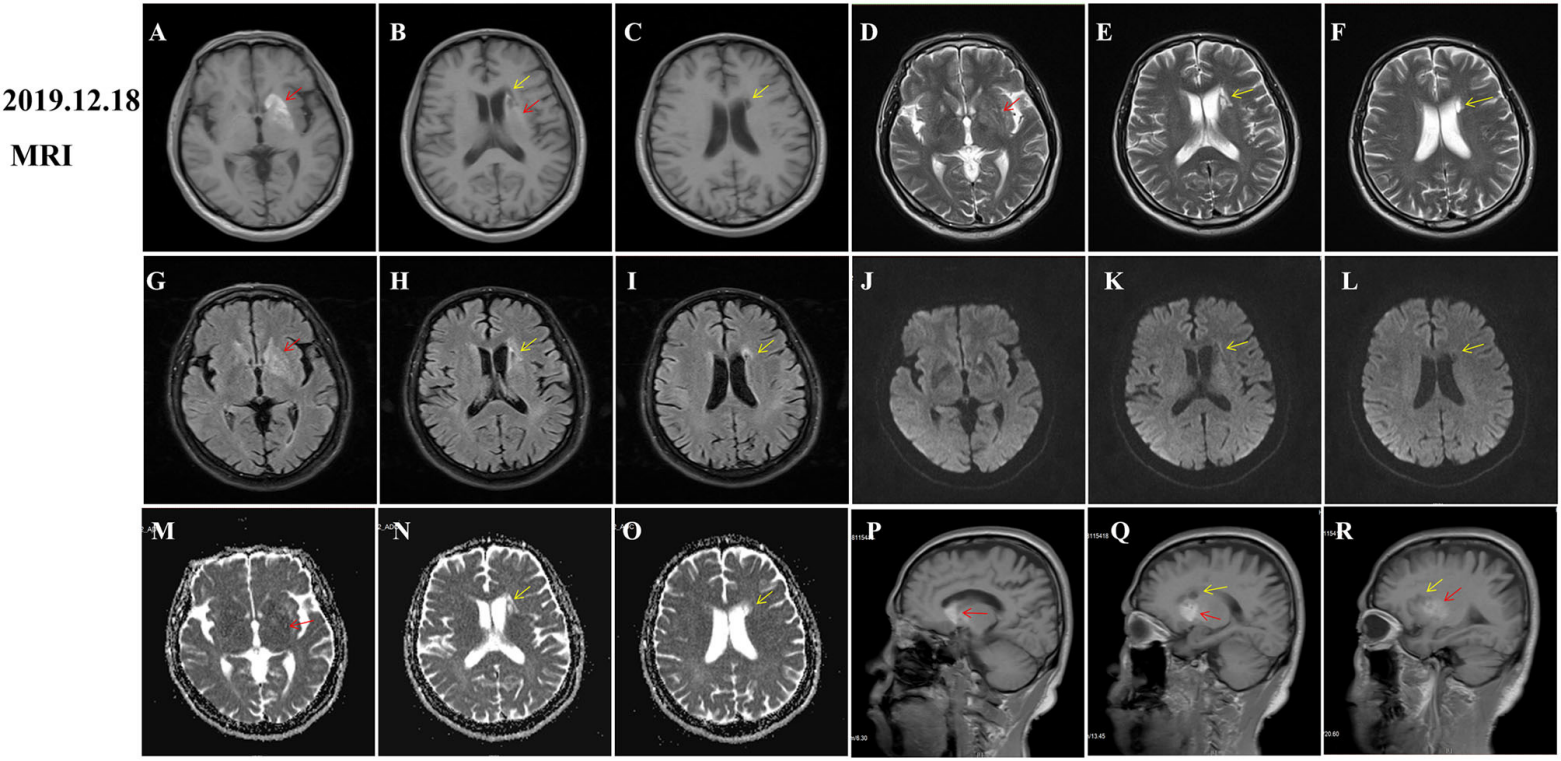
Follow-up magnetic resonance imaging (MRI) of brain 2 months after onset. Diabetic striatopathy (DS)-associated striatal abnormalities were marked by red arrows, while infarction focuses were marked by yellow arrows. DS-associated T1 hyperintensity on the left striatum was obviously extended [(A), coronal image; (P,Q) (R): sagittal images). DS-associated T2 and FLAIR hypointensity changed to slight hyperintensity (D,G). The left striatum showed an equal signal intensity on DWI images (J). DS-associated initial ADC hypointensity changed to equal signal intensity (M). DWI hyperintensity on infarction lesions disappeared (K,L) and ADC hyperintensity increased (N,O). Abnormal T1 hypointensity (B,C), T2 hyperintensity (E,F), and FLAIR hypointensity on the infarction core (H,I), as well as FLAIR hyperintensity around the infarction core (H,I) suggested the formation of softening lesions and secondary gliosis.
